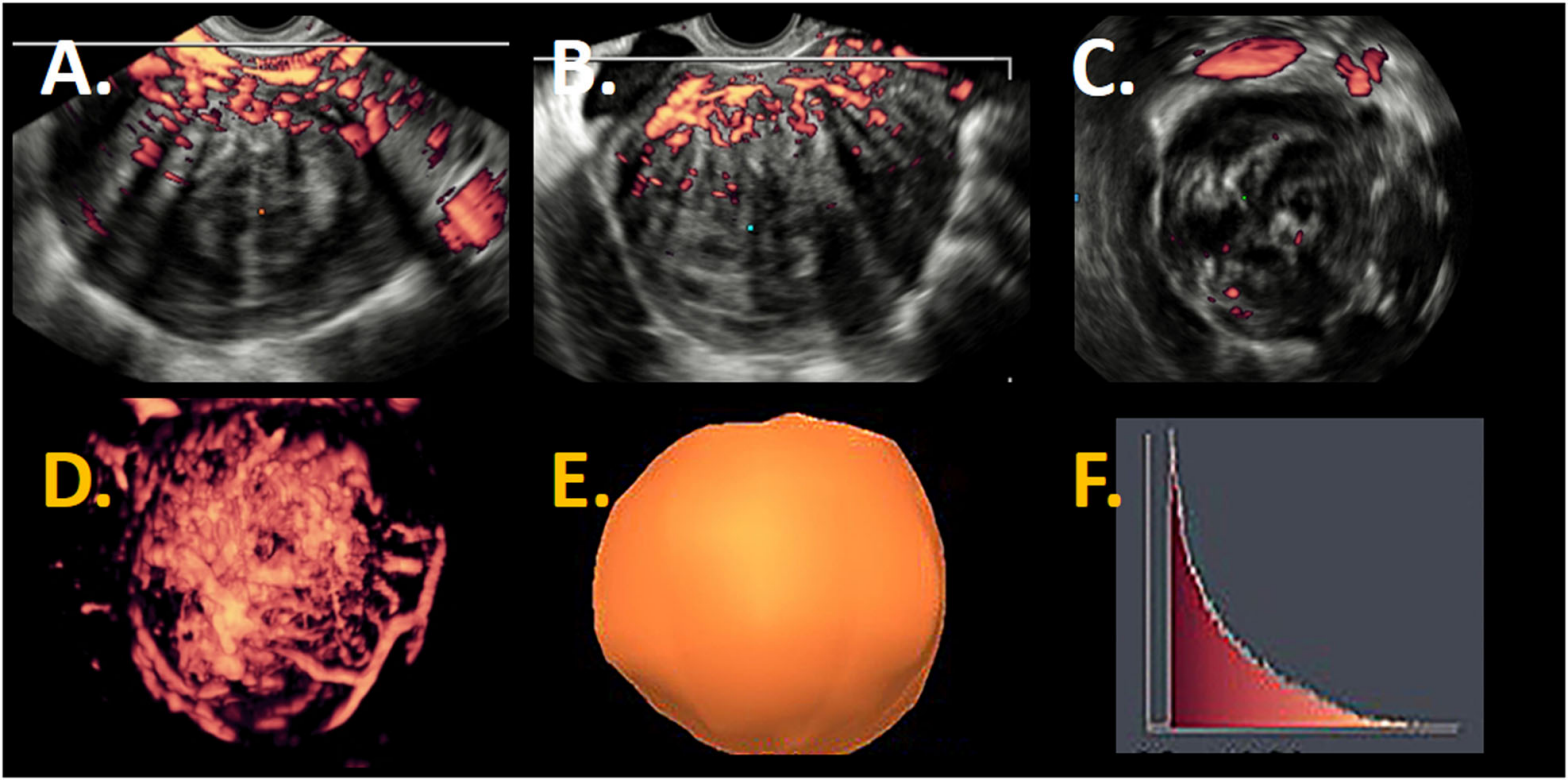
Overview 3D power Doppler images in patient 1. In sagittal view (A), transversal view (B), and coronal view (C). 3D power Doppler reconstruction (D) with a 3D power Doppler volume (E) as result and a histogram (F) as output of 3D power Doppler ultrasound with vascular indices as result
